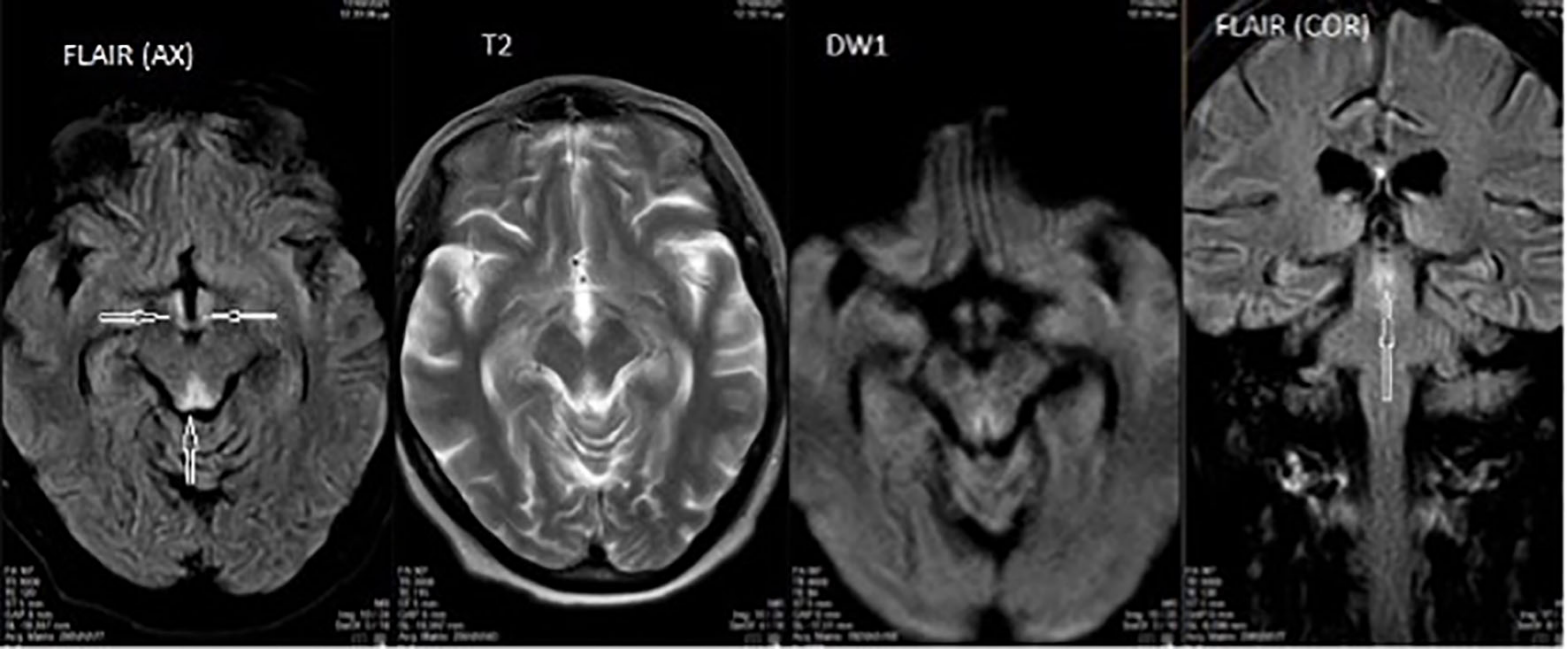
Axial and coronal brain MRI demonstrating T2/FLAIR hyperintensity in the periaqueductal gray matter of the upper pons and midbrain, consistent with findings typically seen in Wernicke’s encephalopathy.
